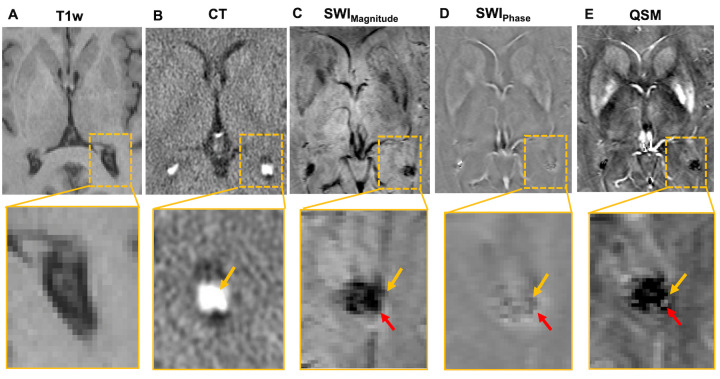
Imaging findings of the dystrophic calcification in the ChP. (A) T1-weighted MRI does not clearly depict the calcification. (B) Computed tomography (CT) reveals hyperattenuating calcifications (yellow arrow), typically interpreted as physiologic change. (C) On the SWI magnitude image, both venous blood (red arrow) and calcification (yellow arrow) appear hypointense; (D) However, on the SWI filtered phase image, calcification (diamagnetic) appears hyperintense, whereas veins (paramagnetic) appear hypointense. (E) On QSM, the ChP calcification (yellow arrow) appears hypointense, while venous blood (red arrow) appears hyperintense.
